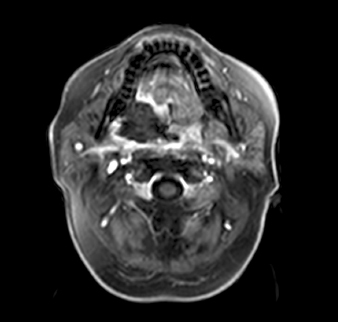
Postablation contrast enhanced MRI image, ablation zone encompassing the tumour.
